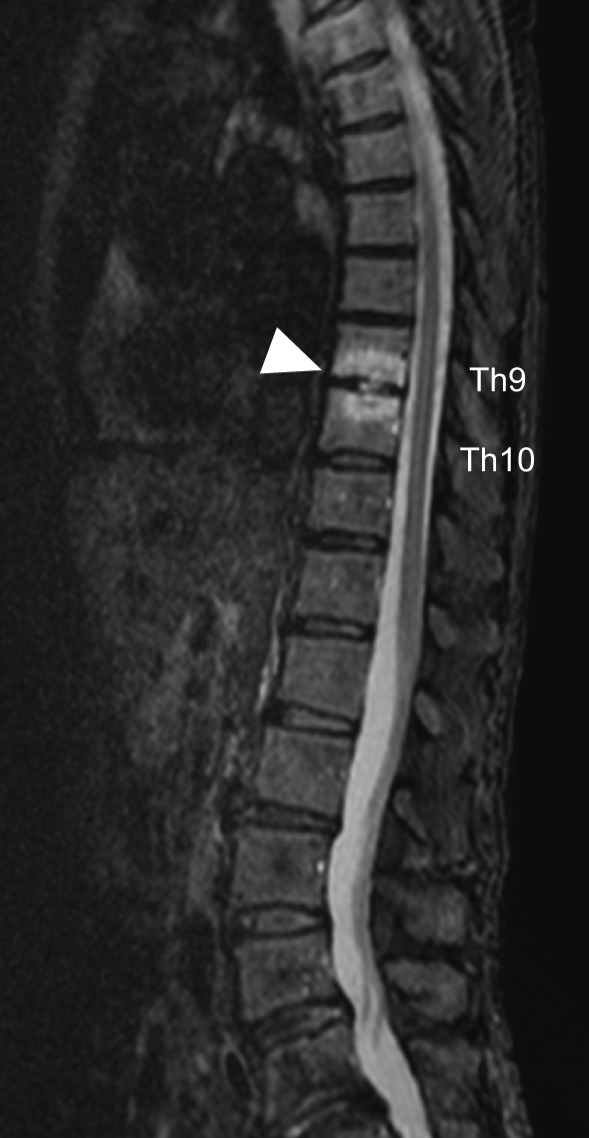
Magnetic resonance imaging of the spine. Short T1 inversion recovery reveals a high‐signal area in the T9–10 disk and T9–10 vertebral body (arrow head)
